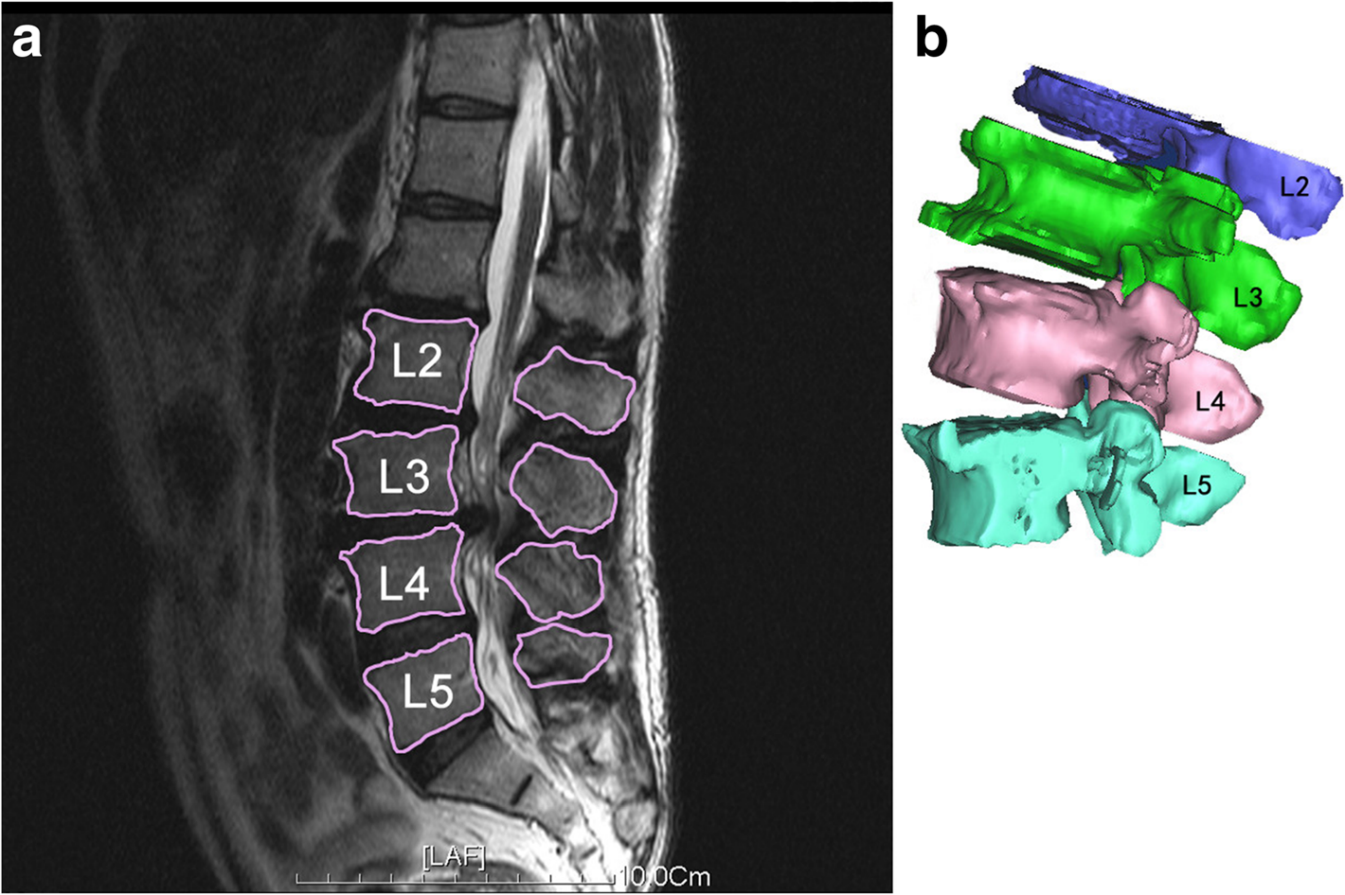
a MR image of human lumbar spine of DDD patients. b 3D anatomical vertebral model from L2 to L5 constructed using the MR images. Local coordinate systems at the tip of spinous process were used to calculate the relative 6DOF kinematics of the proximal spinous process with respect to distal spinous process
